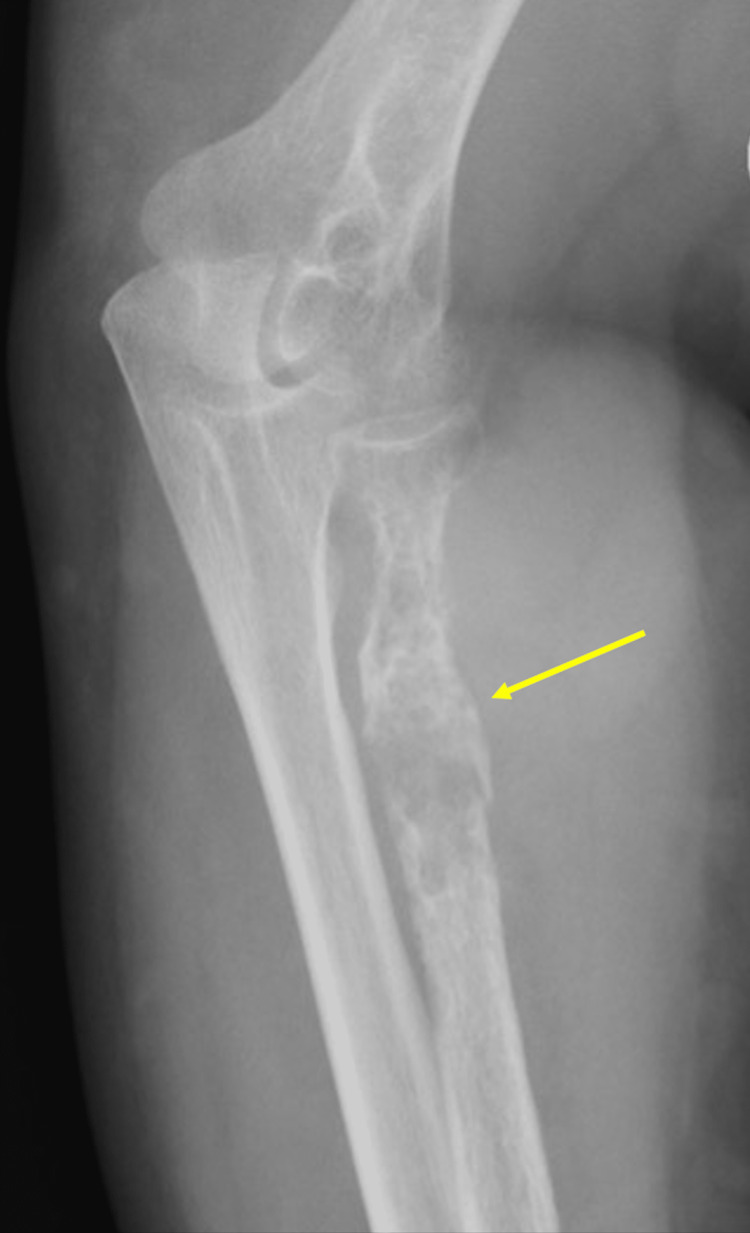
Lateral X-ray of the left elbow and proximal forearm showing aggressive bone erosion
